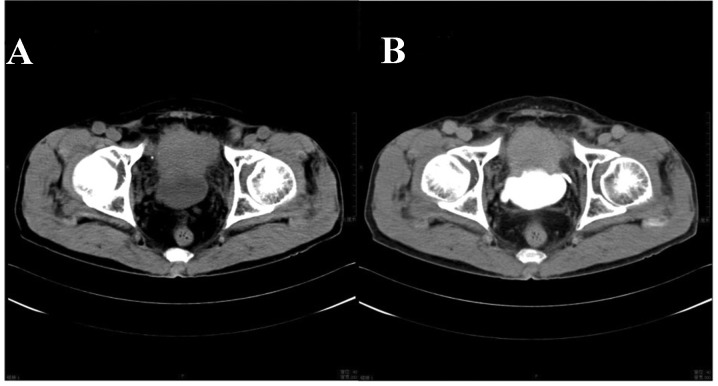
(A) Unenhanced computed tomography scan showing ~6×5-cm soft-tissue shadows measuring 44 HU in the front bladder wall. Irregular filling defects can be observed in the bladder. (B) Enhanced computed tomography scan showing poor definition between the prostate and nidus measuring 72 HU.
